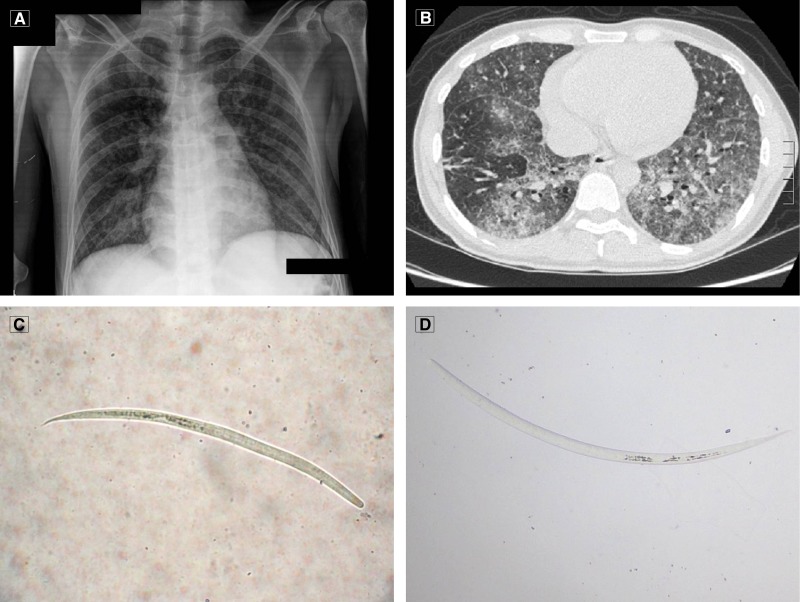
(A) Chest x-rays showing interstitial bilateral infiltrates. (B) High-resolution computed tomography scan showing bilateral ground-glass opacities. (C, D) Bronchoalveolar lavage with multiple *Strongyloides stercoralis* rhabditiform larvae.
